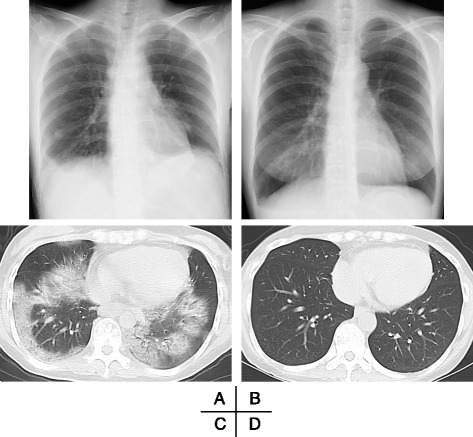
Radiologic findings. A chest X-ray performed on admission with the patient in the supine position showed a nodule-like shadow in the lower lobe of her right lung and reticular shadows in the lower lobes of both lungs (a). A chest X-ray performed on day 10 after admission with the patient in the sitting position showed improvements in the right nodular shadow and reticular shadows of the lower lobes of both lungs (b). Chest computed tomography performed on admission showed subpleural-predominant, nonsegmental ground-glass opacities in the lower lobes of both lungs (c). Chest computed tomography performed on day 19 after admission showed improvements in the ground-glass opacities in both lungs (d)
